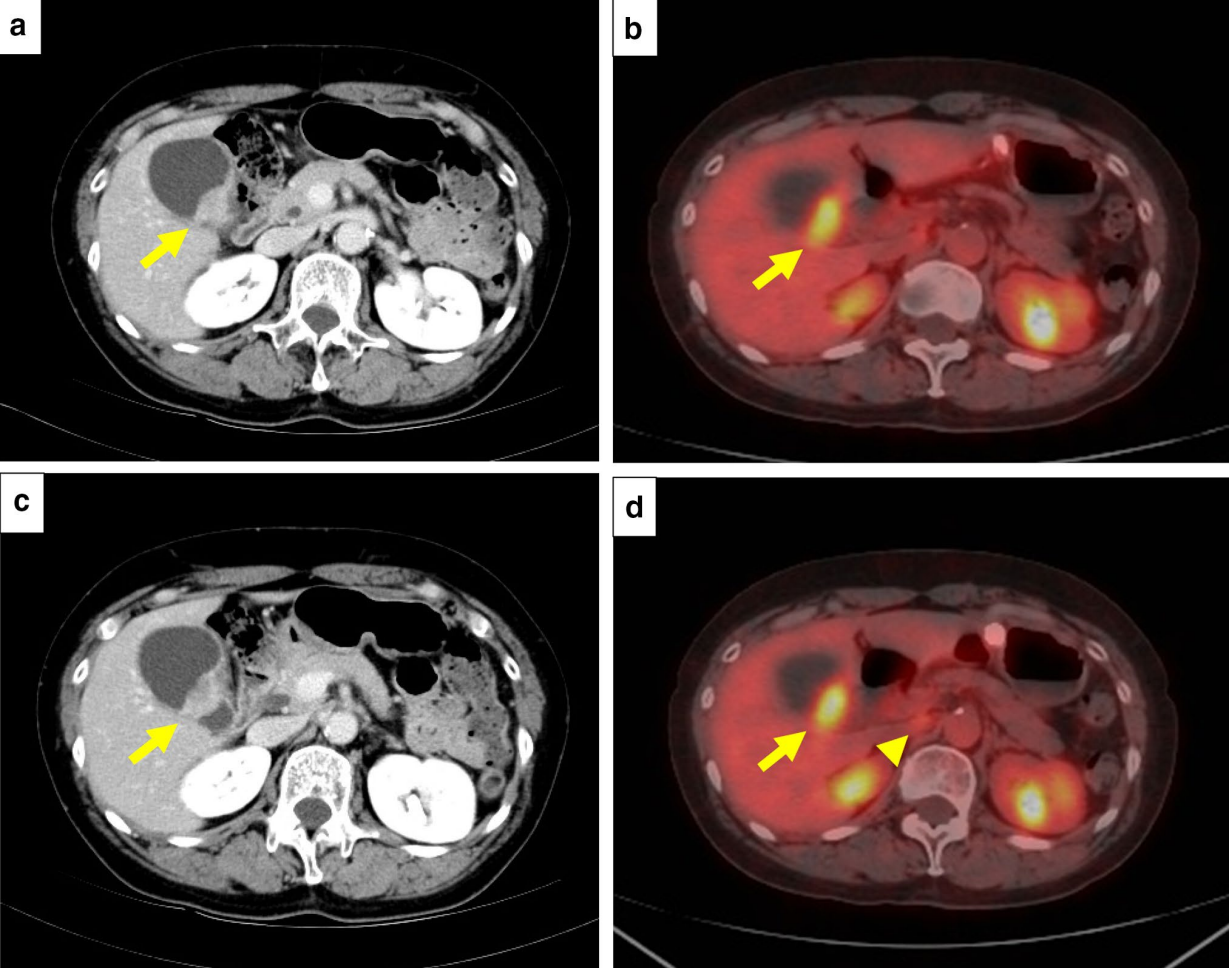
Imaging findings. a and c enhanced abdominal computed tomography (CT) showed a tumorous lesion on the body of the gallbladder (arrow). b
